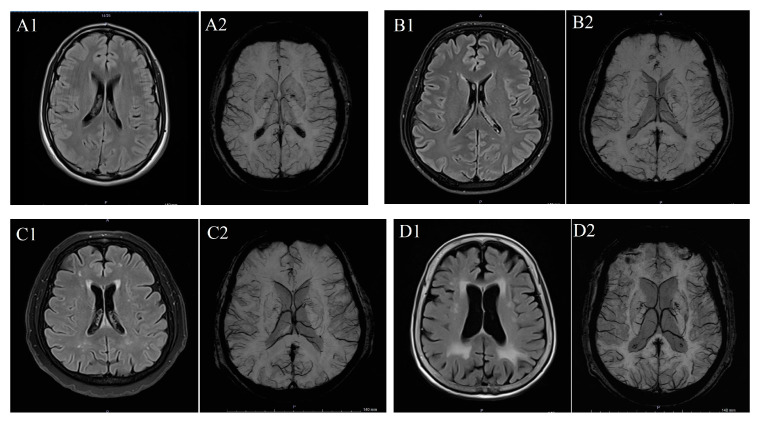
(A) T2 FLAIR (A1) and SW (A2) imaging in a patient with Fazekas 0 case, (B) T2 FLAIR (B1) and SW (B2) imaging in a patient with Fazekas 1 case, (C) T2 FLAIR (C1) and SW (C2) imaging iin a patient with Fazekas 2 case and (D) T2 FLAIR (D1) and SW (D2) imaging in a patient with Fazekas 3 case.
